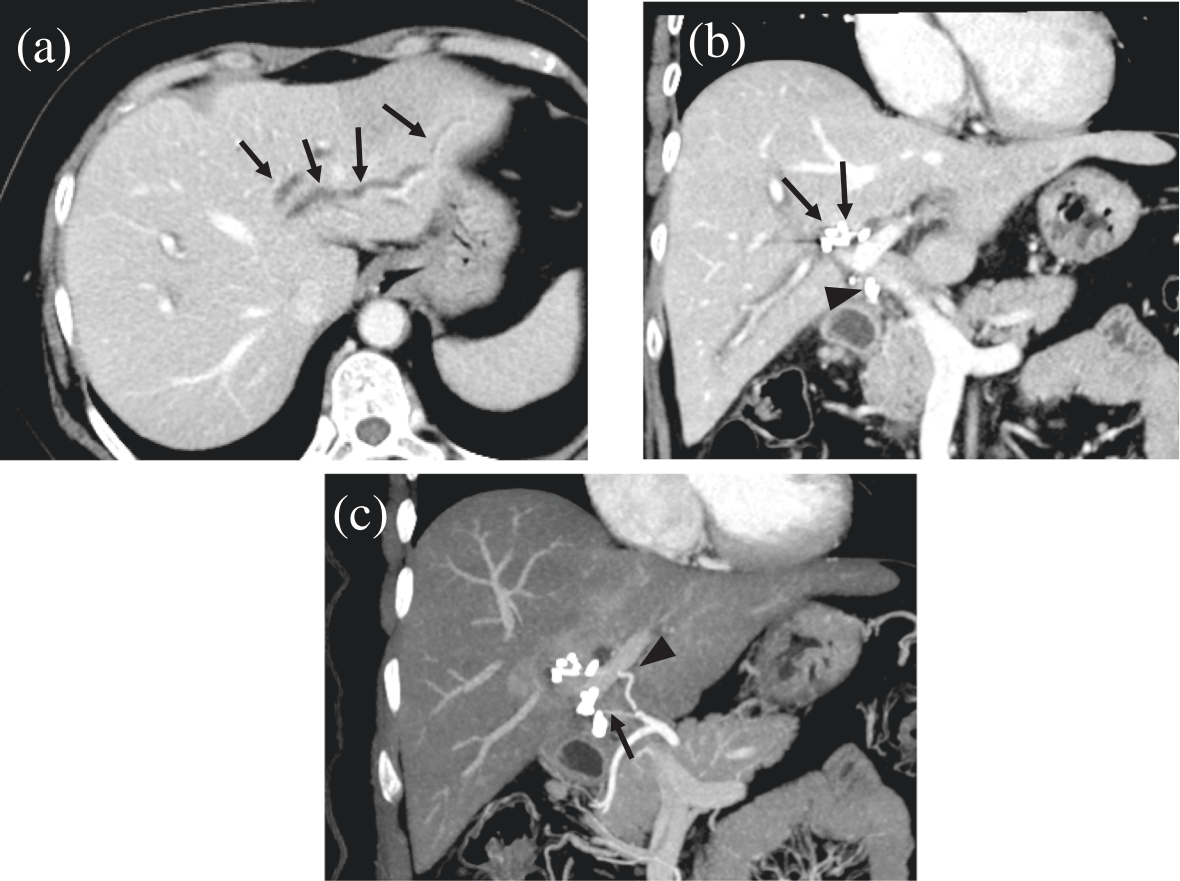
Abdominal enhanced computed tomography (CT) reveals visible expansion of the intrahepatic bile duct of the left lobe of the liver (a
